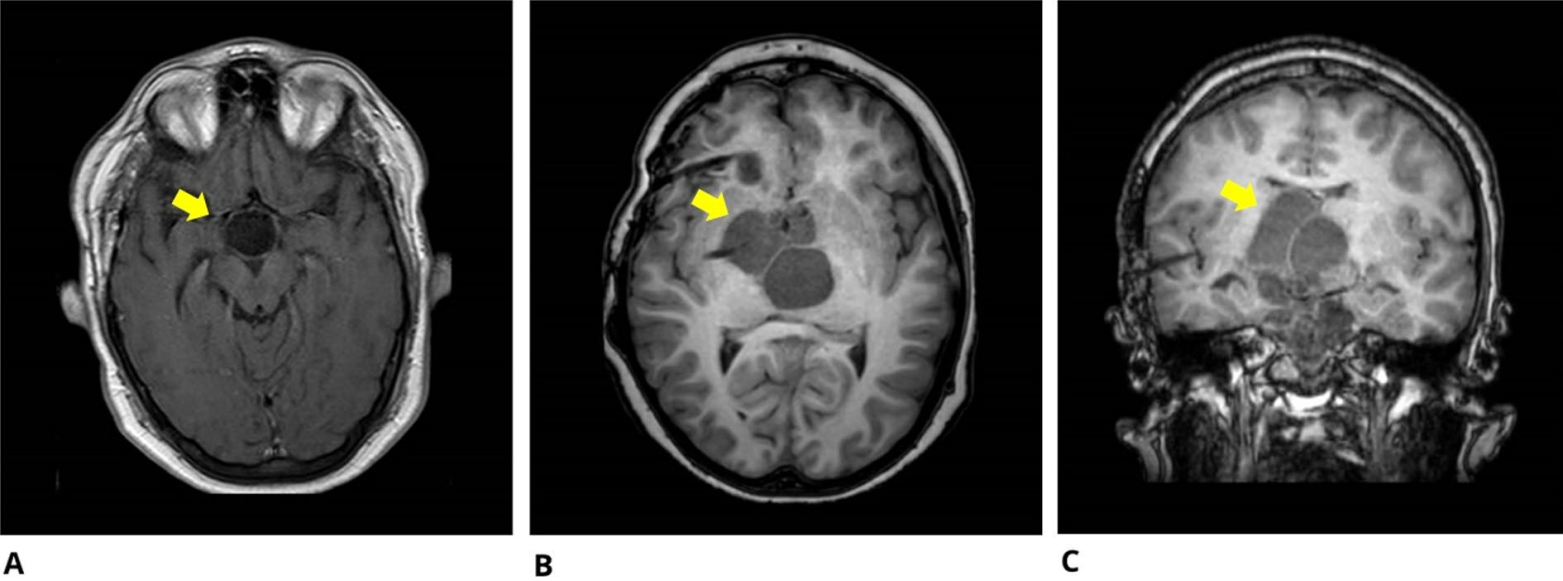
Imaging findings. a T1-weighted brain MR imaging shows the result of craniopharyngioma resection surgery in Patient 1 (yellow arrow). b–c T1-weighted brain MR imaging shows volumetric increase in the post-surgical interpeduncular fossa and right paramedian cysts in Patient 2 (yellow arrow)
